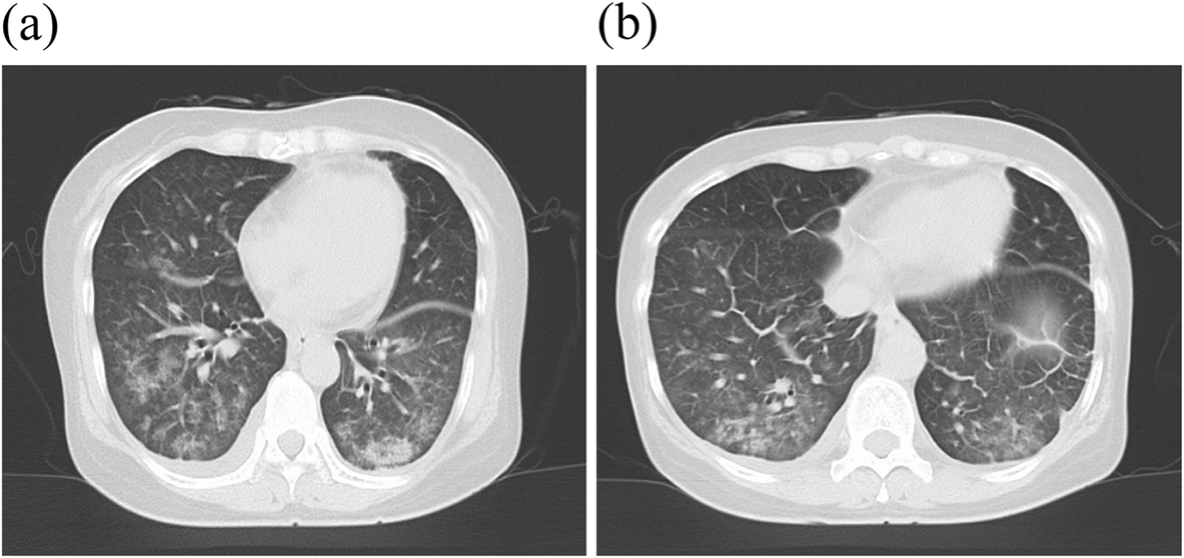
Computed tomography findings sliced at 5.0 mm intervals on June 2, 20XX. Both images have ground-glass opacity in the lower lung fields on both sides. a Image obtained at tube voltage of 120 kV and tube current of 107 mA. b Image obtained at tube voltage of 120 kV and tube current of 153 mA
